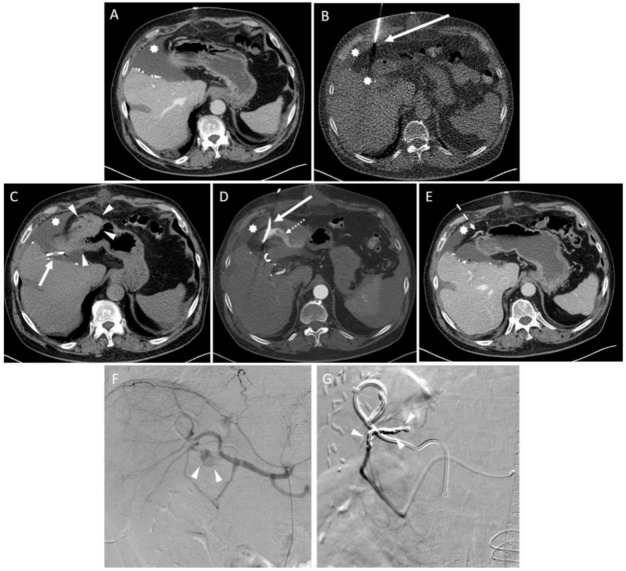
Example of an intervention with complications according to SIR. (A) A 72-year-old male after left side hemihepatectomy due to a cholangiocellular carcinoma. Thirteen days after the operation, the patient presented with fever, epigastric pain and elevated inflammatory blood parameters. CT revealed a large fluid collection in the resection area (star). (B) CT fluoroscopy-guided placement of a 10 F drainage (arrow) within the fluid collection (star). (C) Unenhanced CT post-interventional control scan showed blood collections in the paragastric area and in the right upper quadrant (arrowheads). Arrow: drainage. Star: fluid collection. (D) An additional CT scan with arterial contrast revealed extravasation (dotted arrow). Arrow: drainage. Star: fluid collection. (E) Workup of the incident showed an injury of the right gastroepiploic artery (dashed arrow). The reason was a restless and uncompliant patient. Due to patient movements, the trajectory was not carried out as planned. Star: fluid collection. (F) An immediately performed digital subtraction angiography (DSA) confirmed extravasation of contrast agent from the right gastroepiploic artery (arrowheads). (G) Treatment of the bleeding was performed with an endovascular placement of seven microcoils in a sequence (arrowheads).
